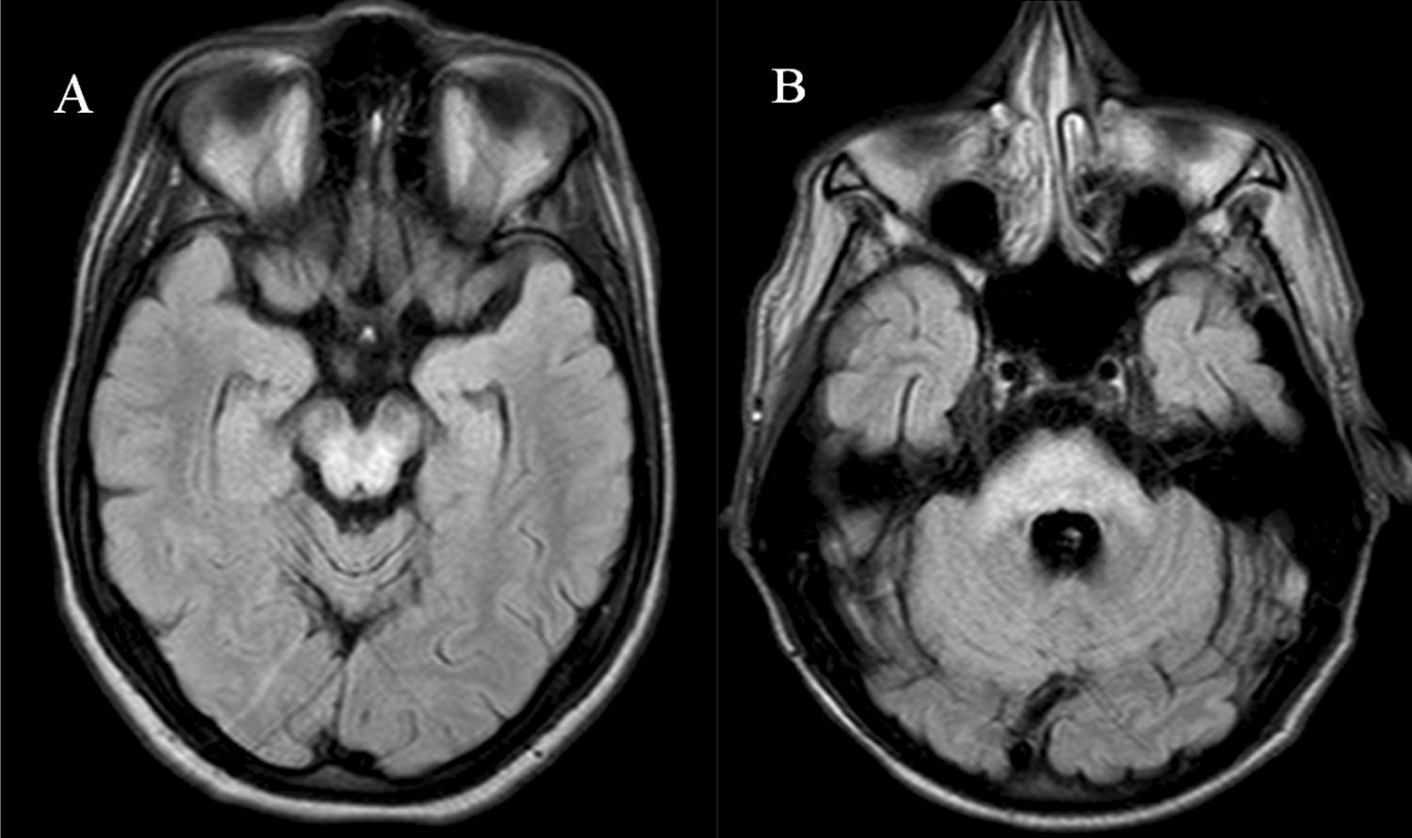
FLAIR image of hyperintensity in: A mesencephalon, B pons
